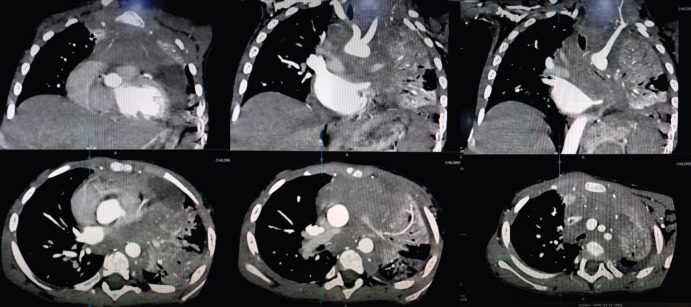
CT scan chest: A soft-tissue density lesion involving the anterior mediastinum extending down to left para-cardiac location. It collectively measures 41 × 50 ×60 mm (anteroposterior × craniocaudal × transverse) and causing compression of the left main stem bronchus and collapse consolidation of almost the whole of the left lung.
